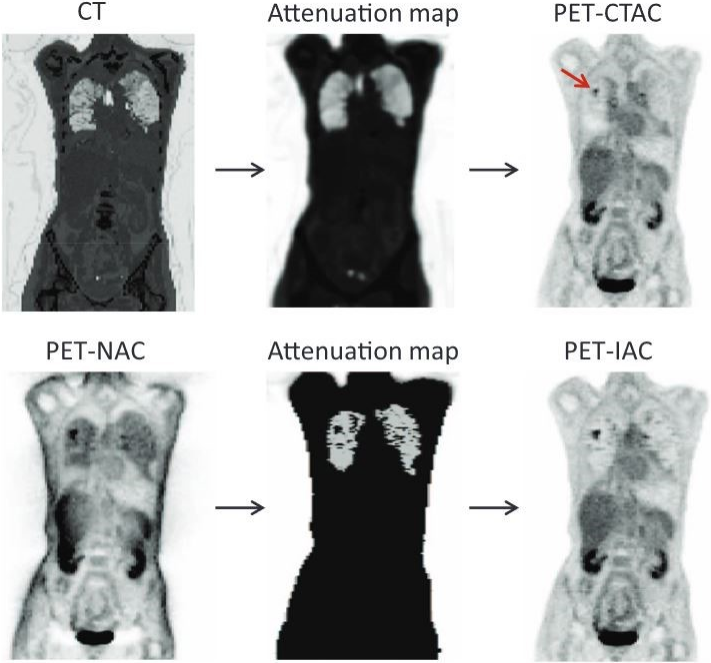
Example computed tomography and nonattenuation‐corrected positron emission tomography segmentation procedures. Adapted from Chang et al.^76^ [Color figure can be viewed at http://wileyonlinelibrary.com]
